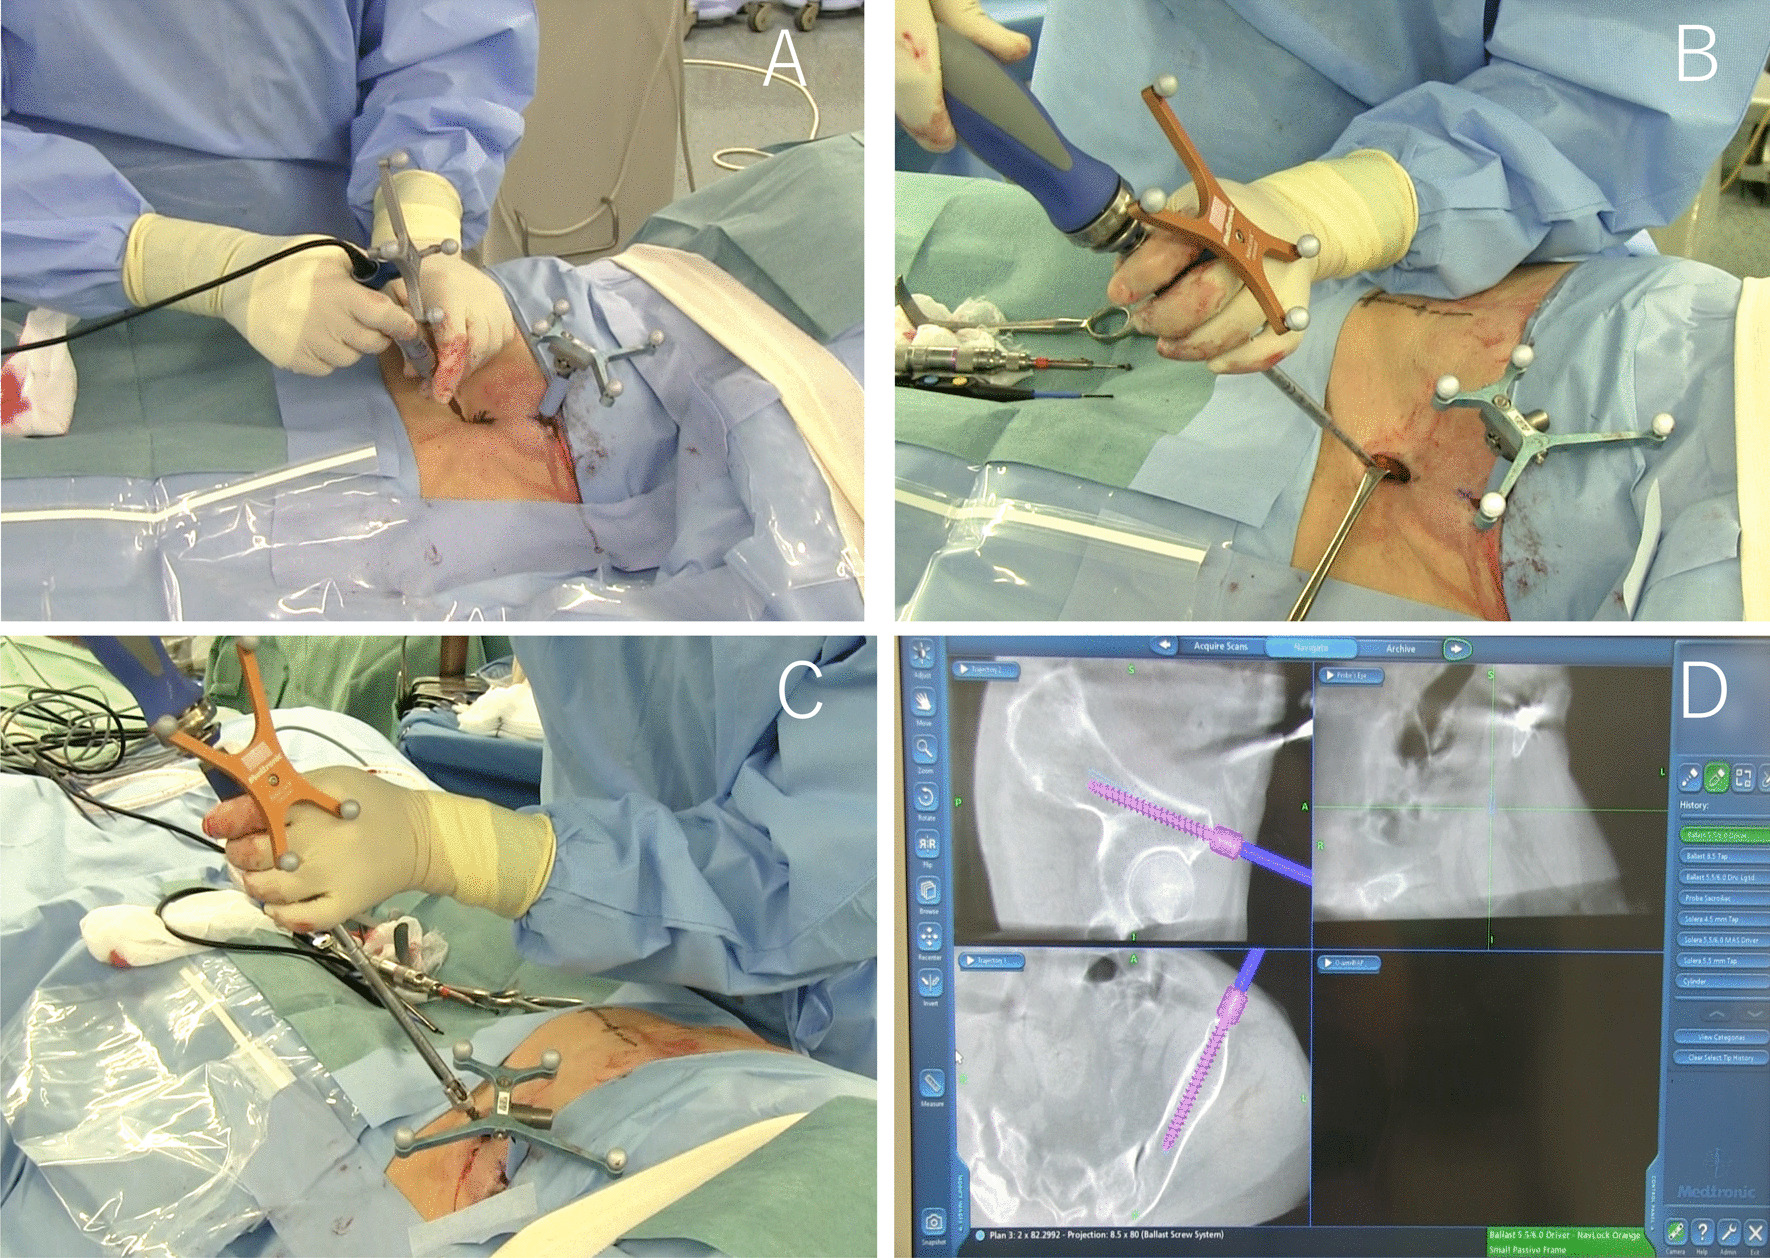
INFIX technique using intraoperative CT navigation. Navigated high-speed burr Stealth–Midas System™ (a). Navigated pedicle tap (b). Navigated CD Horizon® Ballast™ screw (c). StealthStation computer screen projection of a Ballast™ screw being inserted into the iliac crest with a navigated driver (d)
